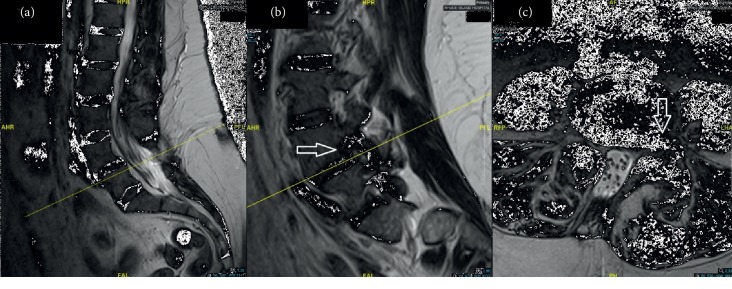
Preoperative lumbar MRI. (a) Midline sagittal T2-weighted MRI demonstrates the L4-5 spondylolisthesis and the prolapsed thecal sac. (b) Left of midline sagittal T2-weighted MRI with foraminal view demonstrates the left L4-5 (arrow) foraminal compression of the exiting L4 nerve. (c) Axial T2-weighted MRI demonstrates left L4-5 far lateral disc herniation compressing the exiting L4 nerve (arrow).
